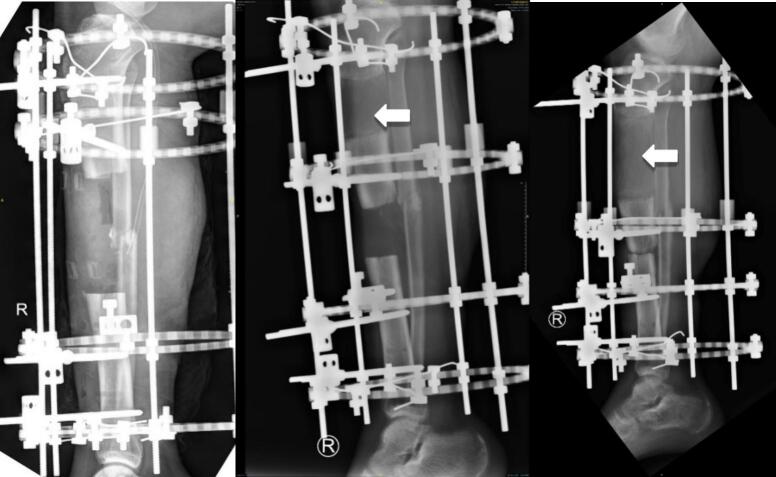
Radiograph images of the leg post Ilizarov application, 3 month follow up, and 6 month follow up, anterioposterior (AP) and right lateral (RL) projections of the right leg. White arrow indicates callus formation.
